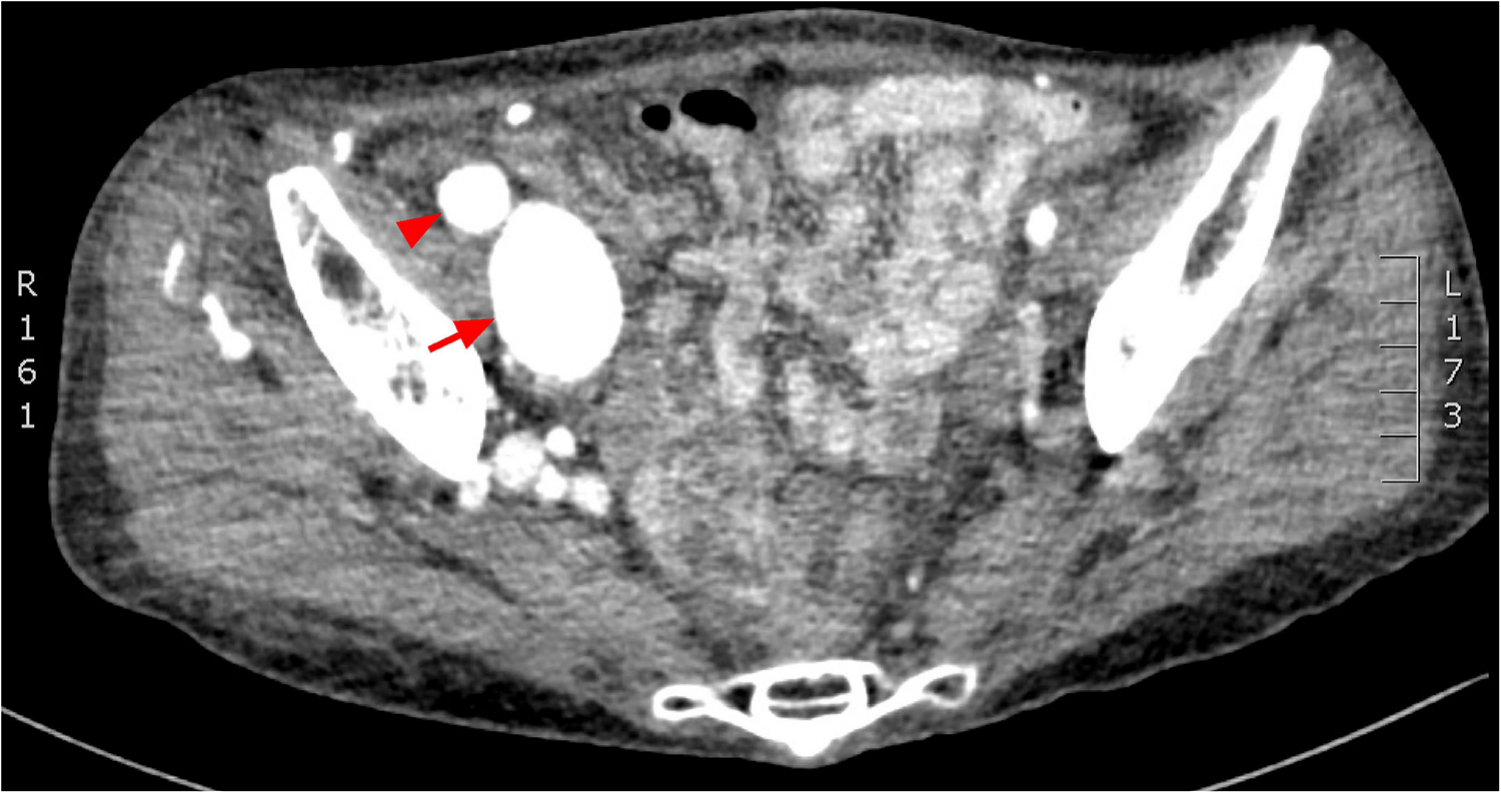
CT angiogprahy: axial section, dilated common iliac artery (arrow head) and vein (arrow).
